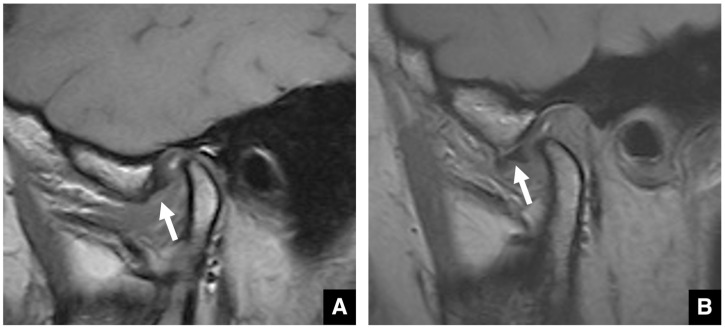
MR images of anterior disc displacement without reduction in the temporomandibular joint. (A) T1-weighted images (TR 1050 ms, TE 18 ms, slice thickness 3.0 mm) show anterior displacement of the articular disc (arrow) in the closed-mouth position and (B) persistent anterior displacement of the disc, without reduction (arrow), in the open-mouth position.
